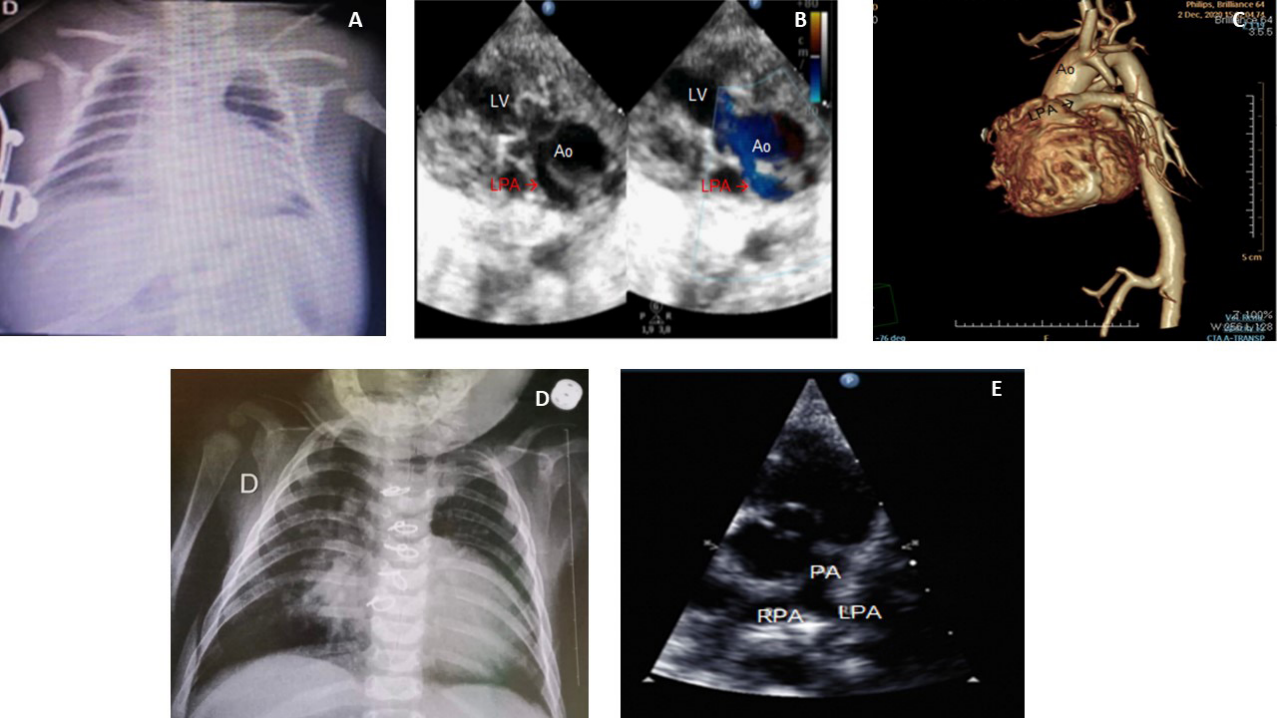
Chest radiography (A), echocardiogram (B), computed tomography
